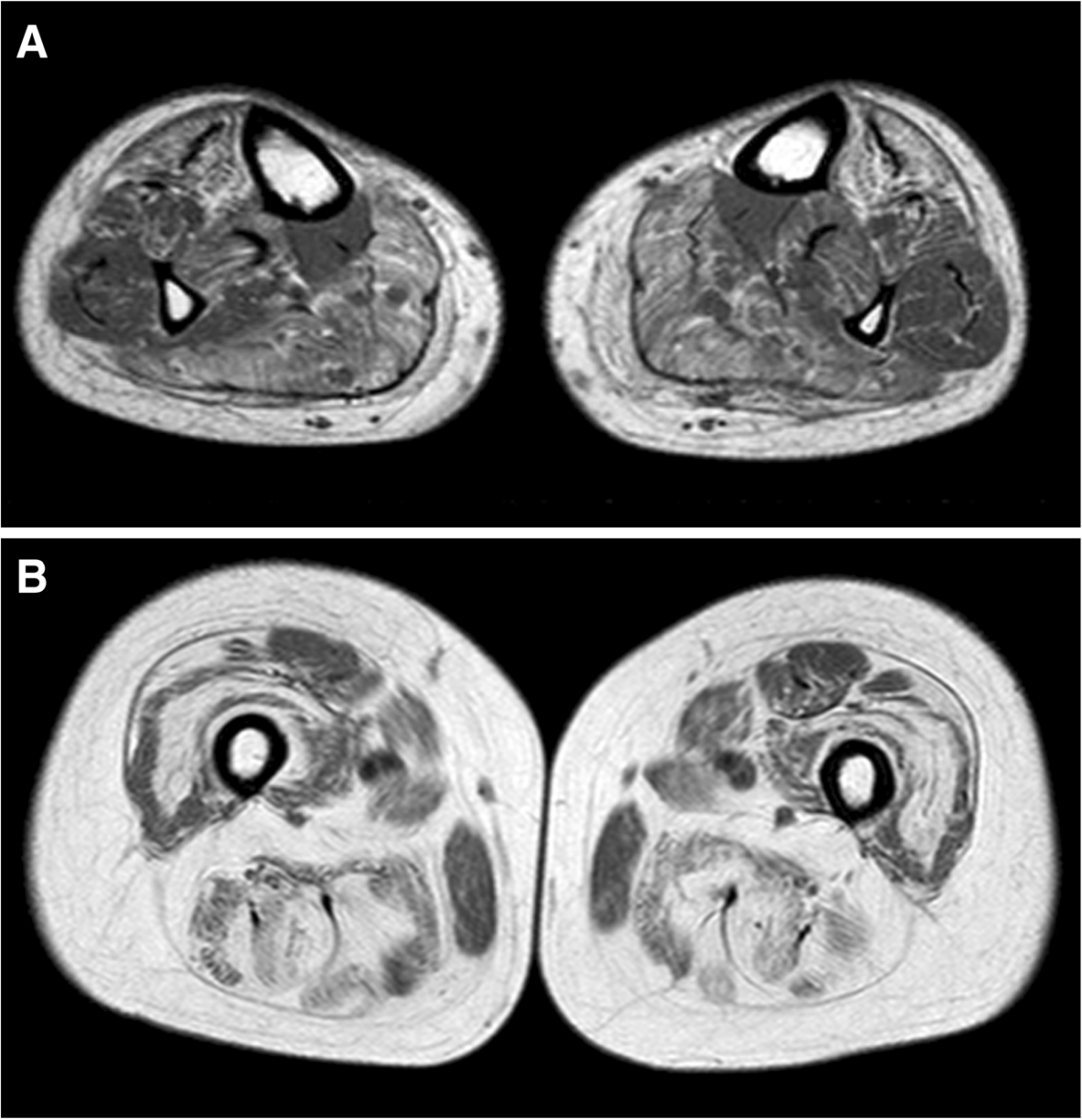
T1-weighted muscle MRI at leg (a) and thigh (b) level. In the leg symmetrical fatty changes are more evident in medial and lateral gastrocnemius and, to a lesser degree, in tibialis anterior muscles (a). In the thigh a diffuse fatty substitution is present, with relative sparing of gracilis and rectus femoris muscles (b)
